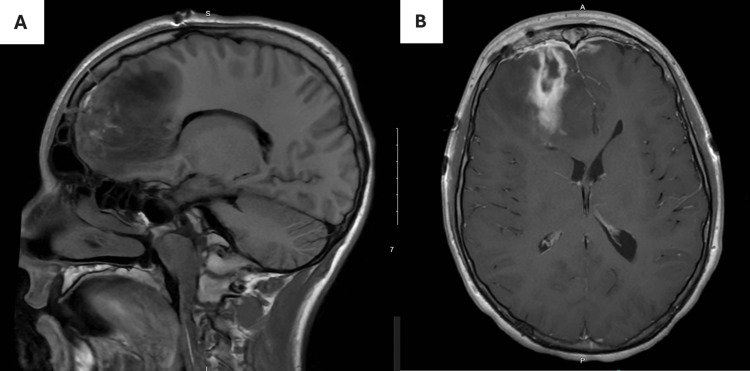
Postoperative MRI of the brain demonstrating residual edema and midline shift The sagittal view (A) and axial view (B) of the brain T1-weighted MRI with contrast six days postoperatively, revealing post-surgical changes, including persistent vasogenic edema with a 4 mm right-to-left midline shift.
